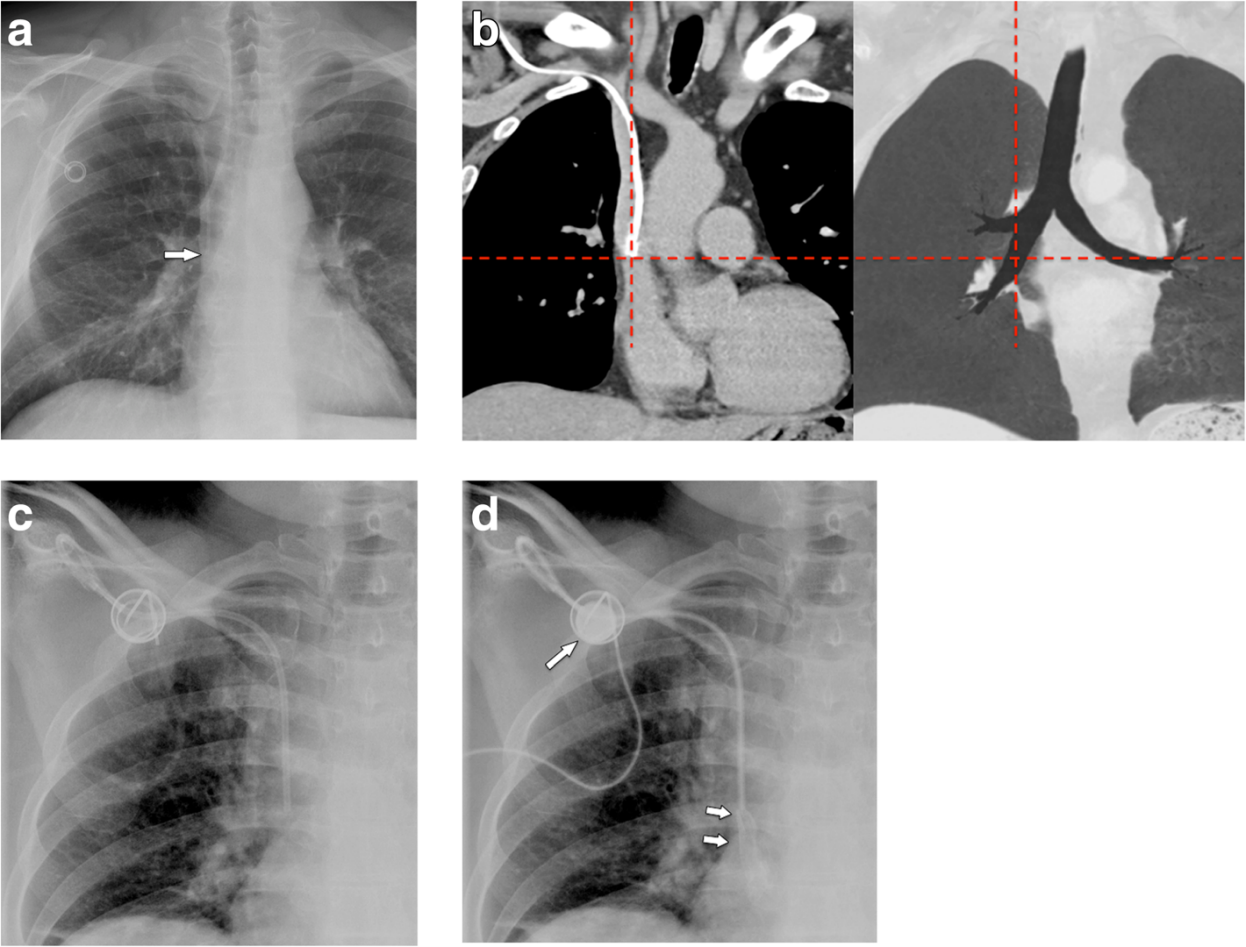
Normal position of implanted port system. a Chest radiograph shows the tip of the venous catheter projecting onto the intersection with the intermediate bronchus, which suggests position in the SVC. b Coronal MDCT reformation (soft tissue window and minimum intensity projection of lung window) shows that intersection of the tip with intermediate bronchus indicates correct position in the SVC. c, d Flow confirmation study (c) before and (d) after injection of contrast. The port chamber is completely opacified (arrow), the venous tube does not show leakage, and contrast material exits freely at the tip flowing in antegrade direction (small arrows)
